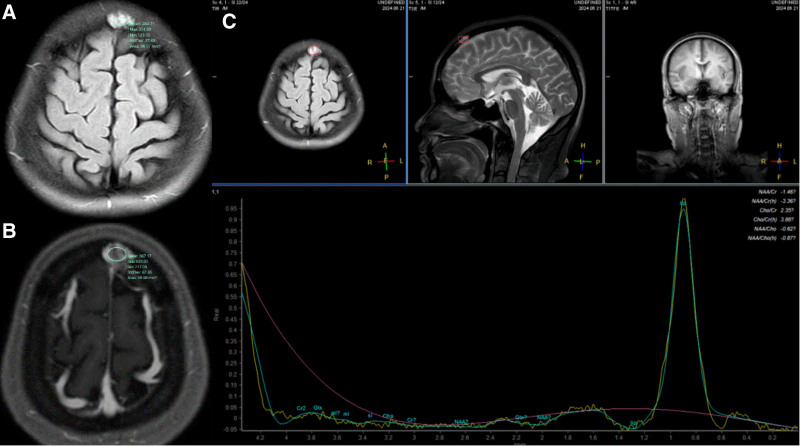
(A and B) The lesion in the cranial bone barrier shows a high signal in T2WI Flair images, and mild to moderate enhancement in T1-weighted MR image; (C) The MRS image no show NAA peak and Cr peak of the lesion, and show the broad Lip peak.
